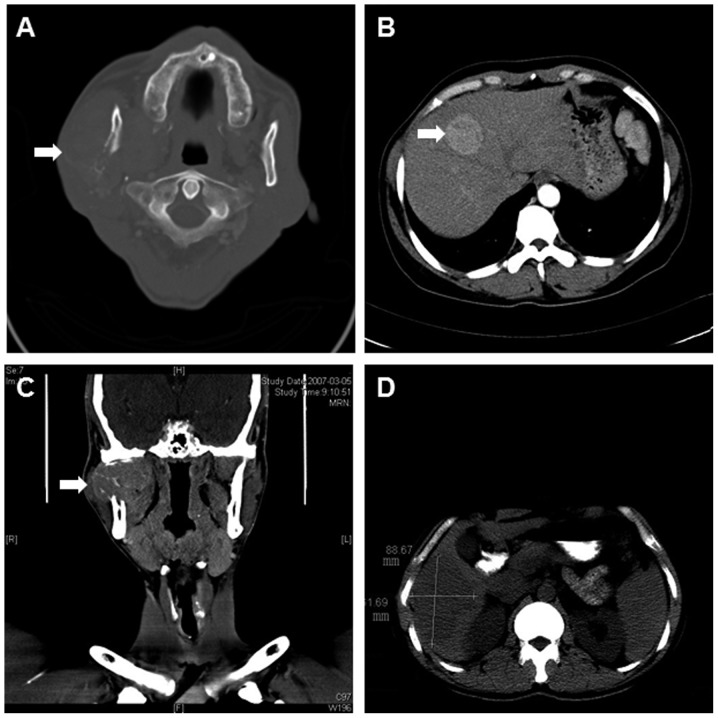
(A and B) Case one: (A) Plain computed tomography scan showing the tumor and osteolytic lesions in the right mandibular ramus. (B) The primary tumor of the hepatocellular carcinoma was identified in the medial segment of the left liver lobe following surgery. (C-D) Case two: (C) Coronal scan showing the tumor in the right mandible, with extension to the cranial base. (D) Primary tumor, 88×78 mm in size and with uneven density, identified in the right liver lobe following surgery.
